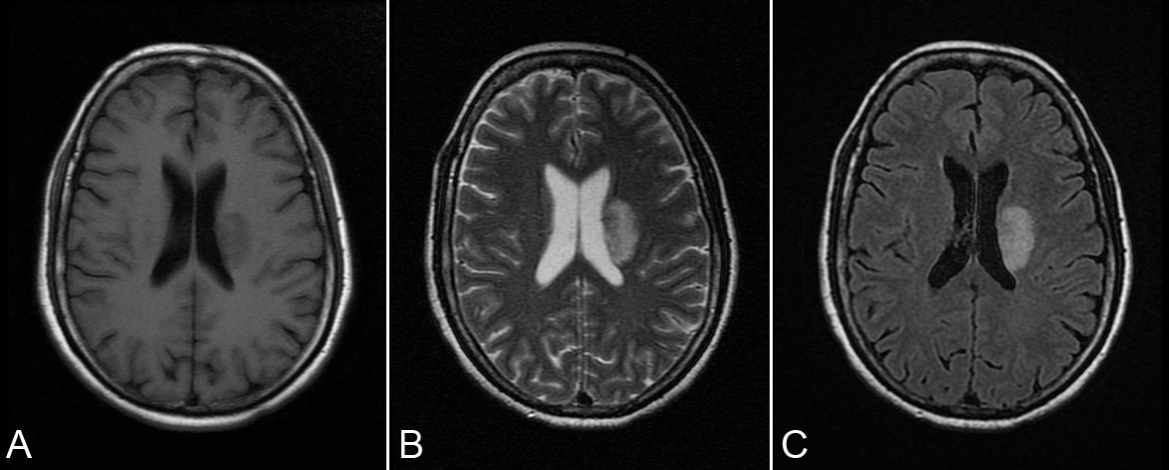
T1-FLAIR, T2-weighted and T2-FLAIR images from a patient show focal infarct at left corona radiate. (A) T1-FLAIR obtained 2 days after stroke show a hypointense area at left corona radiate. (B) T2-weighted image and (C) T2-FLAIR images obtained at W1 show a hyperintense area at left corona radiate.
